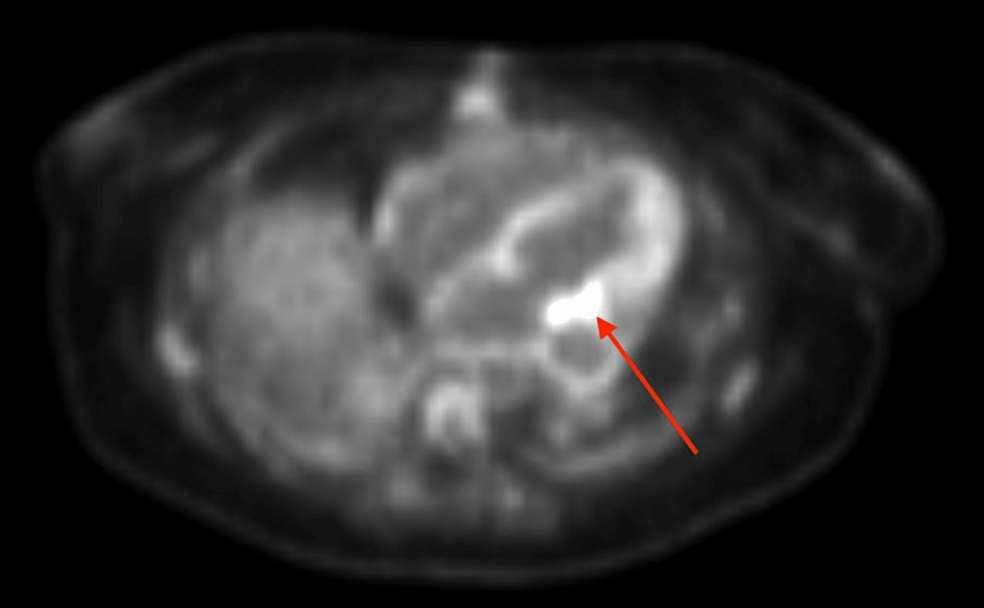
Positron emission tomography scan. The positron emission tomography scan conducted after echocardiography identified a mass along the superior aspect of the mitral annulus and left atrial appendage clip. The red arrow indicates increased uptake of fluorodeoxyglucose, which indicated an infectious source for mitral endocarditis.
